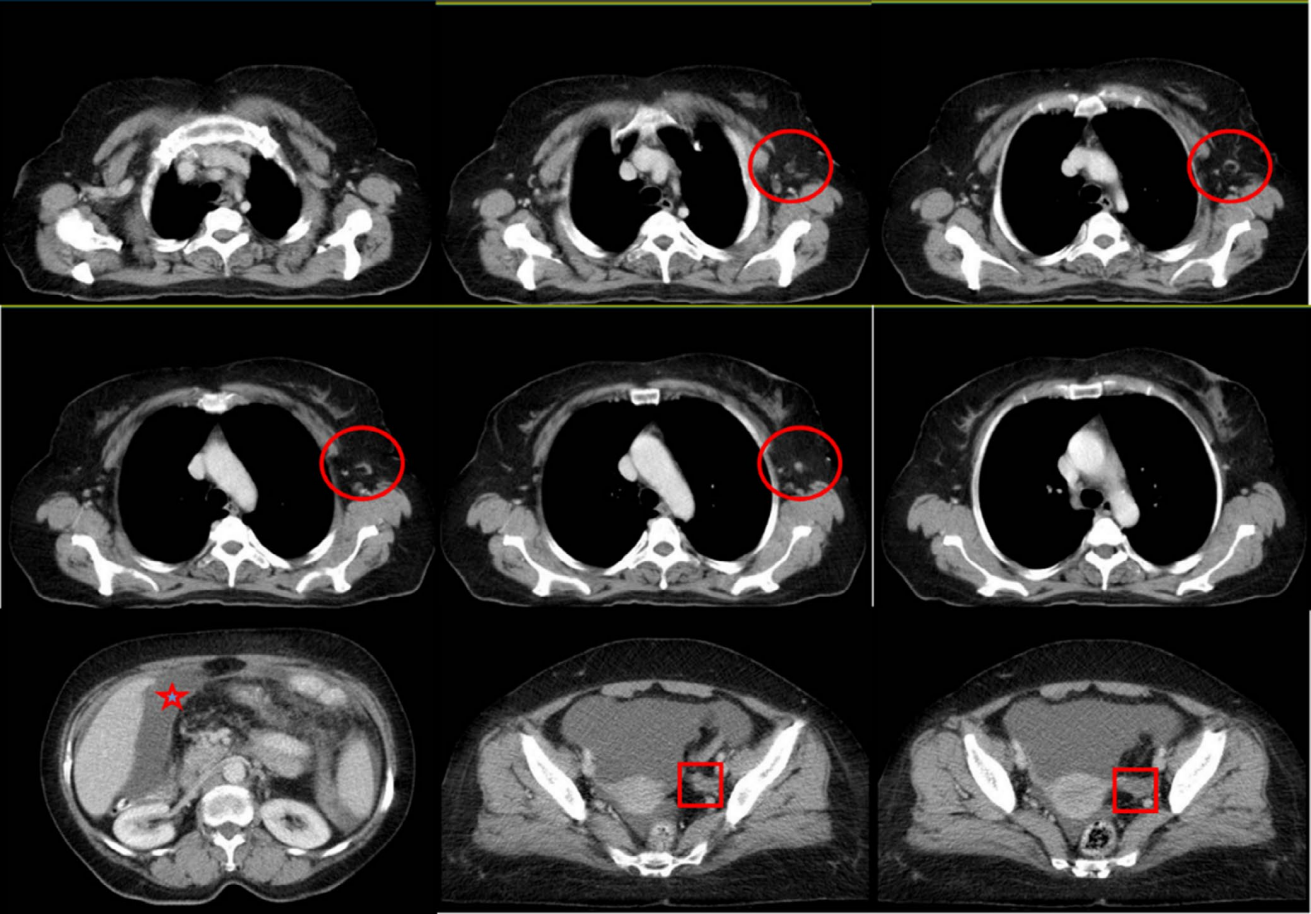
Whole‐body computed tomography (CT) scan showed lymphadenopathies of left axillary region (circle) along with moderate ascites (star) and left ovarian mass (square)
